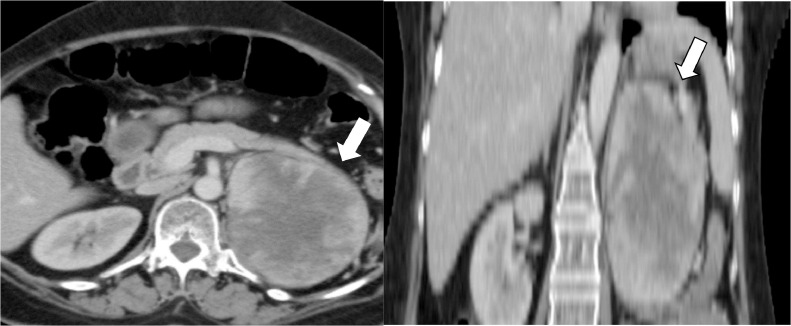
Five-month follow-up CT scan showing interval increase in the size of soft tissue mass (white arrow, axial, and coronal view) in the left nephrectomy bed.
